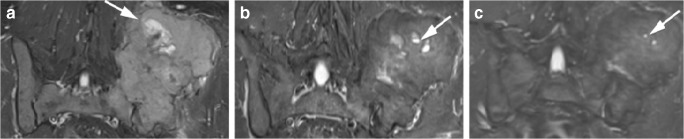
A 36-year-old man presented with an enlarging mass originating from the left ilium. MRI at presentation (a), after 12 weeks on denosumab (b) and after 20 weeks on denosumab treatment (c). a Coronal T1 TIRM MR image at baseline shows large cystic foci in the left iliac GCTB (arrow). b Coronal T1 TIRM MR image after 12 weeks on treatment demonstrates that the lesion appears smaller and of lower signal intensity, with decreased size of the cystic components (arrow). c Coronal T1 TIRM MR image after 20 weeks on treatment shows further reduction in size and signal intensity of the GCTB and the cysts are barely visible (arrow)
